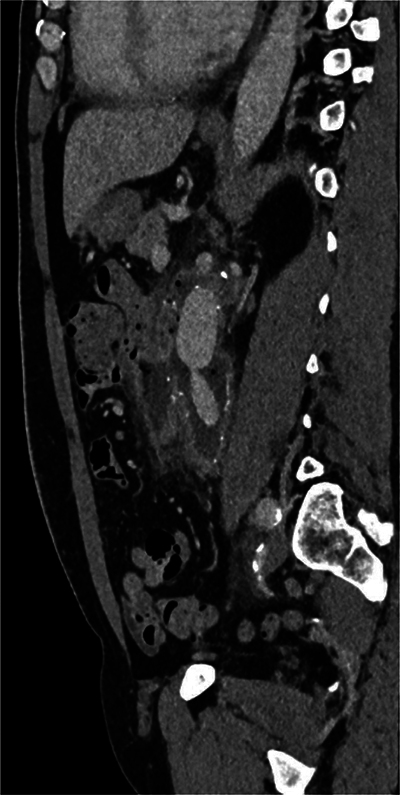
Sagital CT scan showing the close contact between a jejunal loop and the aneurysmal sac.
